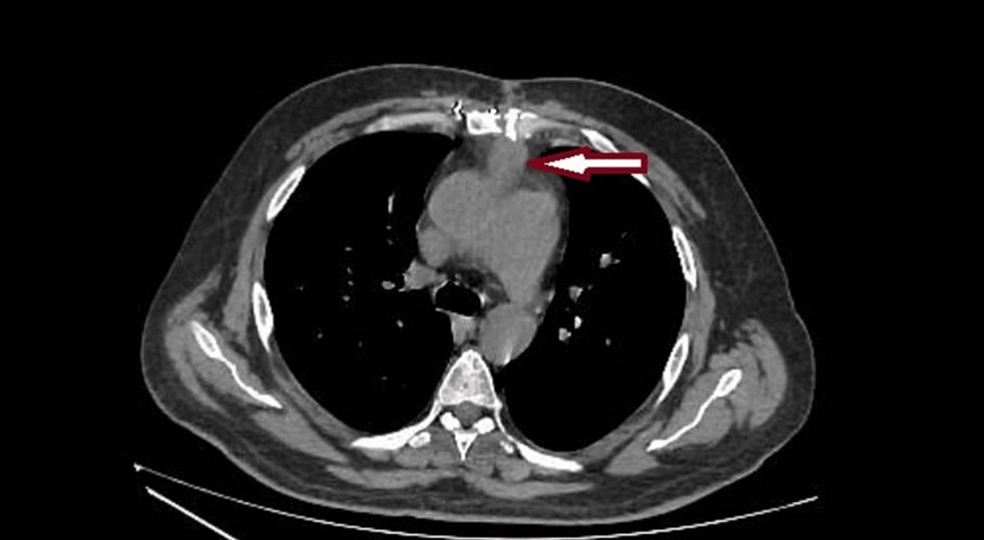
Chest CT demonstrating well-defined hypodensity (arrow) in the anterior mediastinum close to the anterior aspect of the ascending aorta as well as the posterior aspect of the sternum, with an appearance suggestive of loculated fluid
